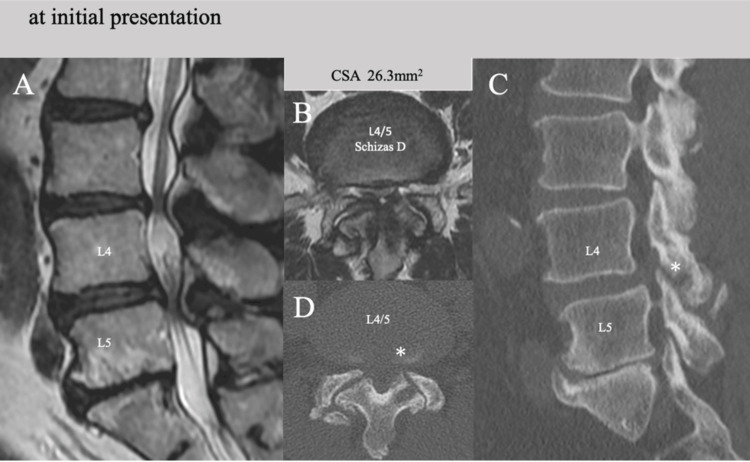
Preoperative MRI and CT images of Case 2 MRI and CT images of a 74-year-old man (Case 2) showing (A, B) moderate to severe canal stenosis at four disc-levels (Schizas D at L4/5) with (C, D) L4 degenerative spondylolisthesis and osteophyte formation of L5 superior facet (asterisks). CSA: cross-sectional spinal canal area
